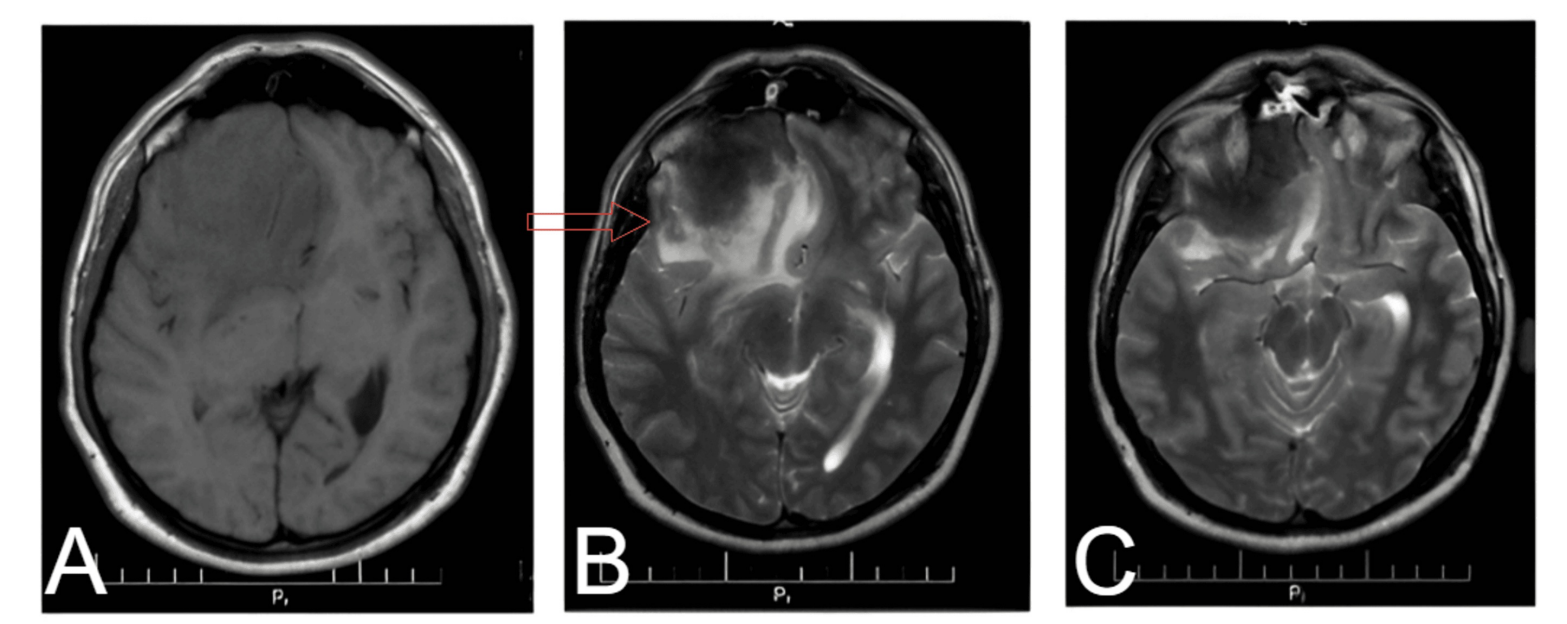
Axial MRI images demonstrate a frontal skull base lesion with associated T2 hyperintensity and perilesional edema, suggestive of a granulomatous pathology. (A) There is a lesion in the frontal skull base. (B) The lesion is hyperintense on T2-weighted imaging, indicating edema or increased water content in the surrounding brain parenchyma. (C) The lesion's involvement of adjacent structures, including the skull base and potential compression of the optic nerves, is more clearly seen.
